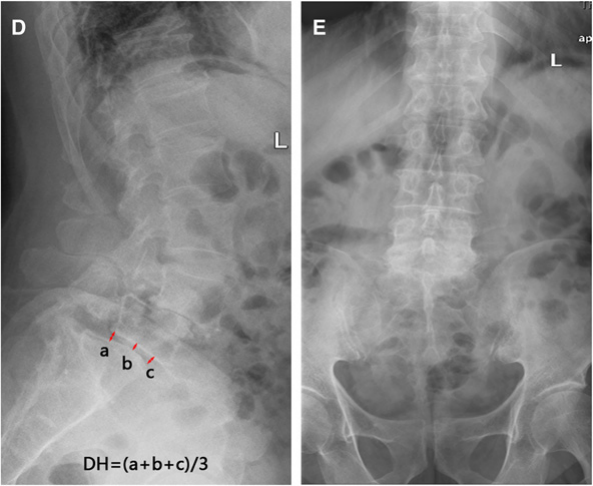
D, E MRI, CT, X-ray show double-level spondylolisthesis at L4 and L5, in MRI and CT show the corresponding segment of the nerve compression. Preoperative lateral lumbar spine. Slipping of L4 and L5 resulting in II spondylolisthesis, and bilateral spondylolysis at L4 and L5
